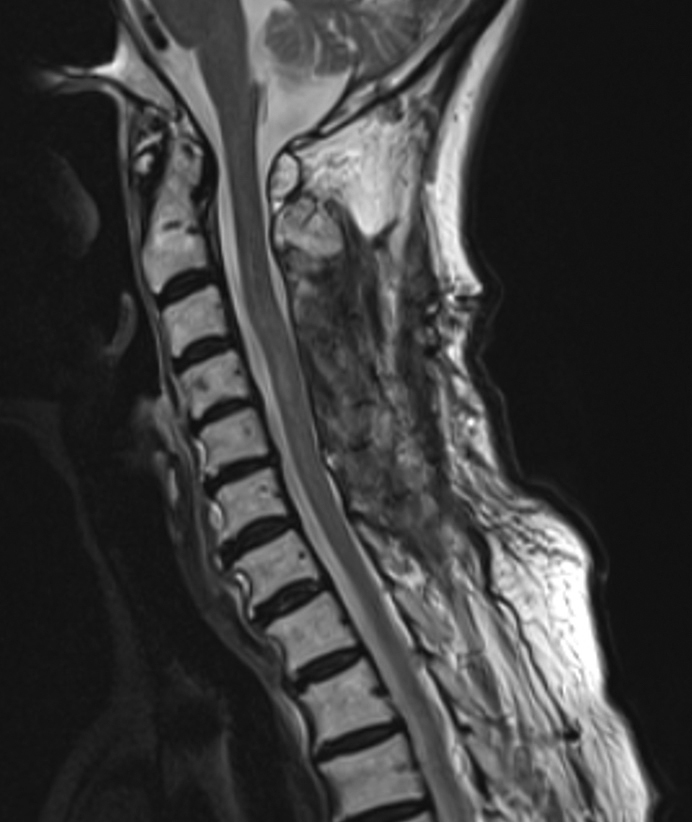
Two months after surgery, the MRI revealed complete resolution of the abscess with resolution of surrounding edema. (T2WI Sagittal). MRI: magnetic resonance imaging; T2WI: T2 weighted image.
